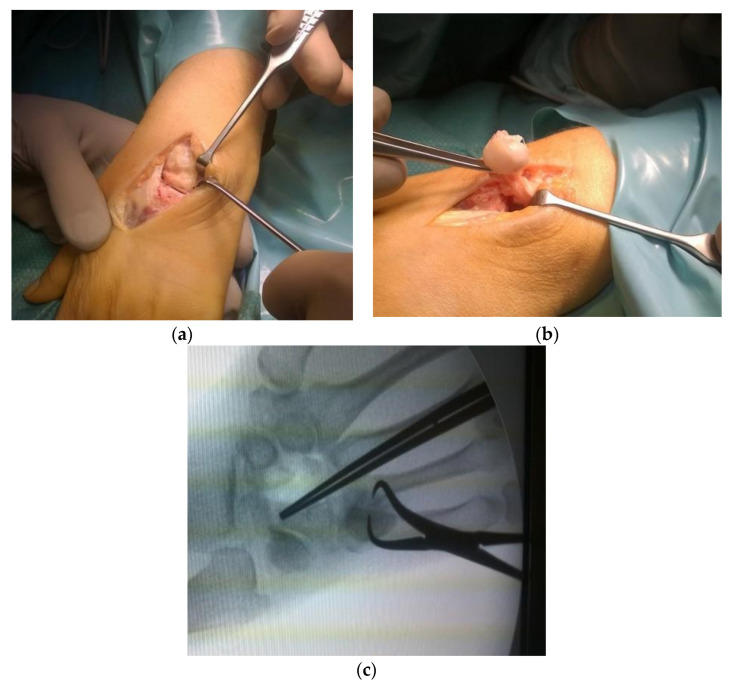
Photographs were obtained during surgery in a patient undergoing vascularized capitate transposition and capitate osteotomy combined with the vascularized bone transfer (modified Granner’s method). (a) A view of the anatomy of the radiolunate joint fully exhibited using a dorsal surgical approach; (b) Vascularised capitate graft being harvested; (c) X-ray of the wrist after lunate excision and transposition of the capitate.
